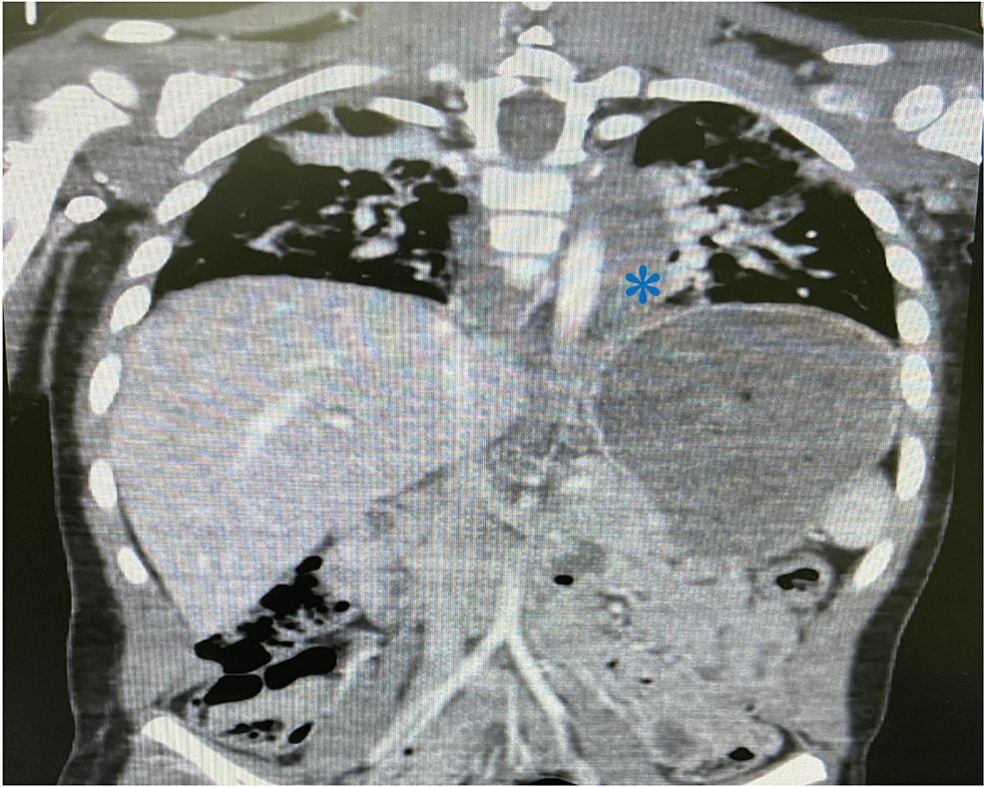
CT chest showing grade III aortic pseudoaneurysm (asterisk )
